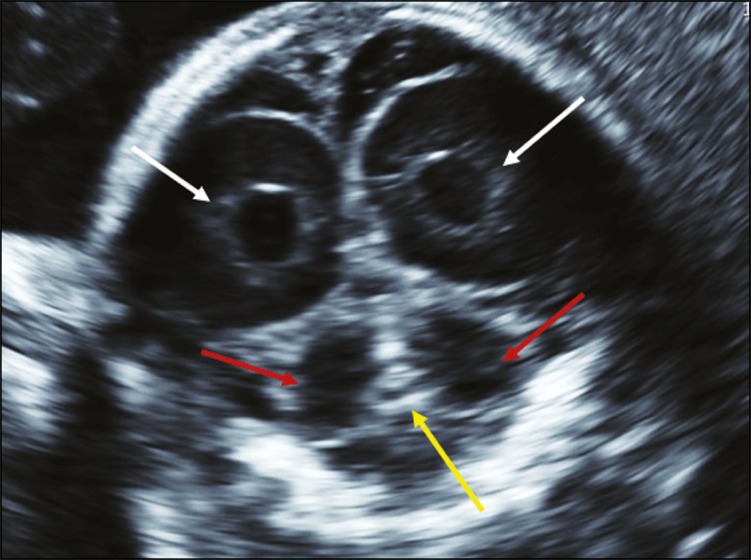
Coronal ultrasound of the transcerebellar plane, evaluating the cerebellum (shape, contours, and foliations of the cerebellar hemispheres); the vermis (more highly echogenic structure located between the two cerebellar hemispheres, yellow arrow). Coronal imaging is very important for differentiating between the cerebellar hemispheres and the vermis.
